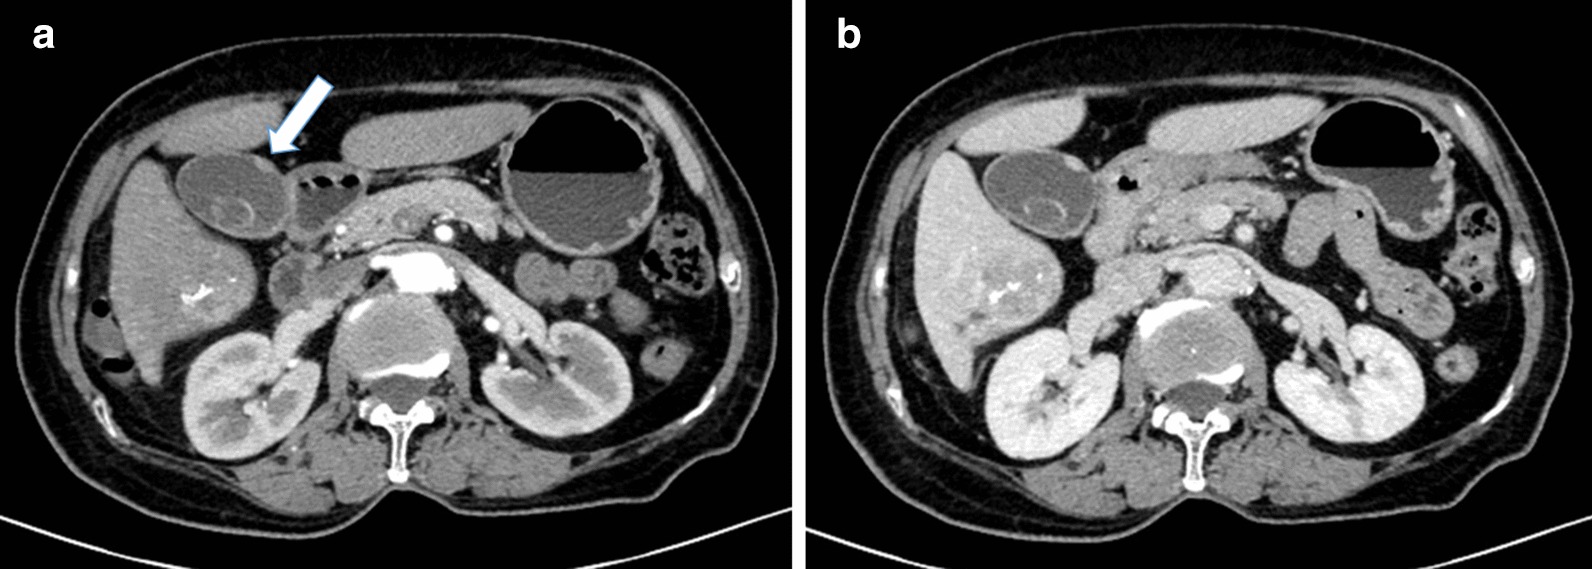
Abdominal CT images after TACE to observe the gallbladder mainly. a Arterial phase (white arrow indicating nodular thickening in the gallbladder wall), b venous phase
